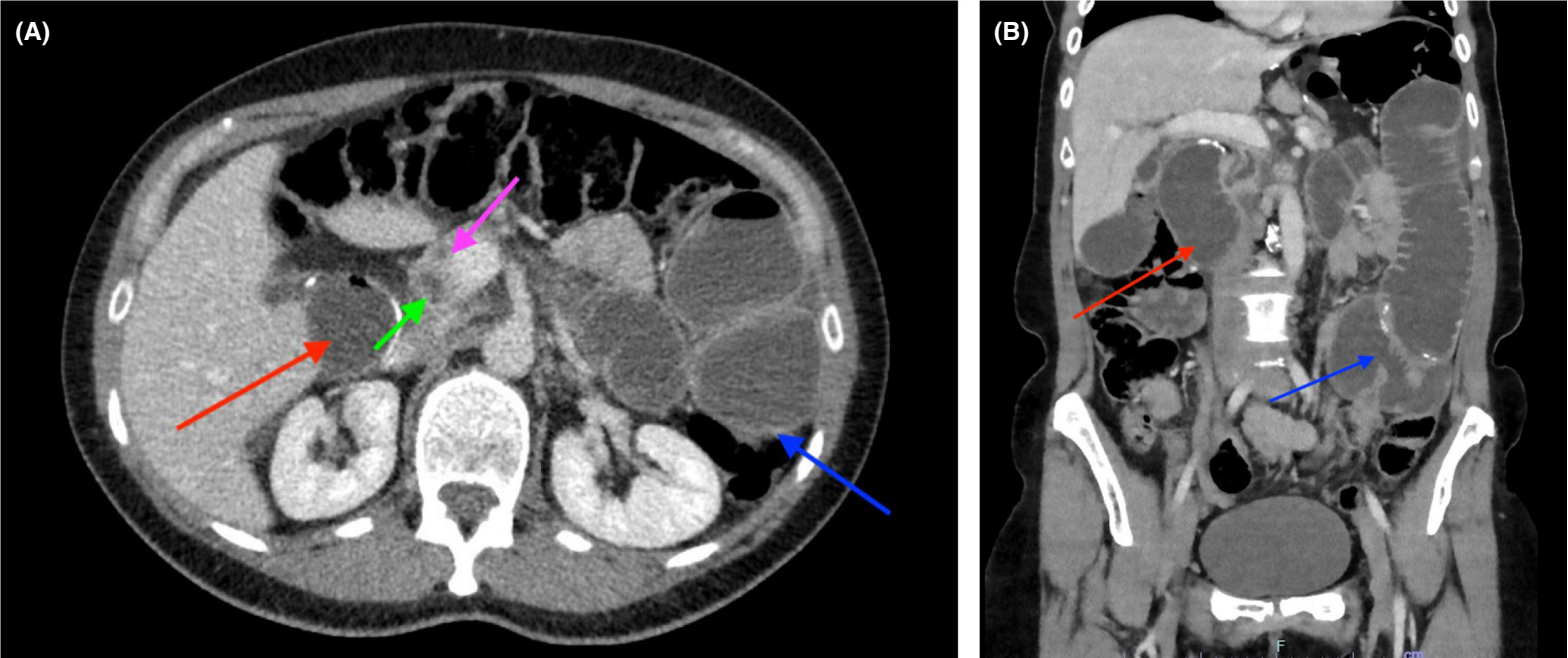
(A) Axial computed tomography of abdomen and pelvis showing a proximal small bowel obstruction, involving the efferent and afferent loops of the Roux‐en‐Y gastric bypass. Red: afferent limb; blue: efferent limb; green: common bile duct; pink: pancreatic duct. (B) Coronal computed tomography of abdomen and pelvis showing a focal transition point within the mid‐abdomen involving the small bowel, distal to the entero‐enterostomy (blue arrow). Dilated afferent limb (red arrow).
